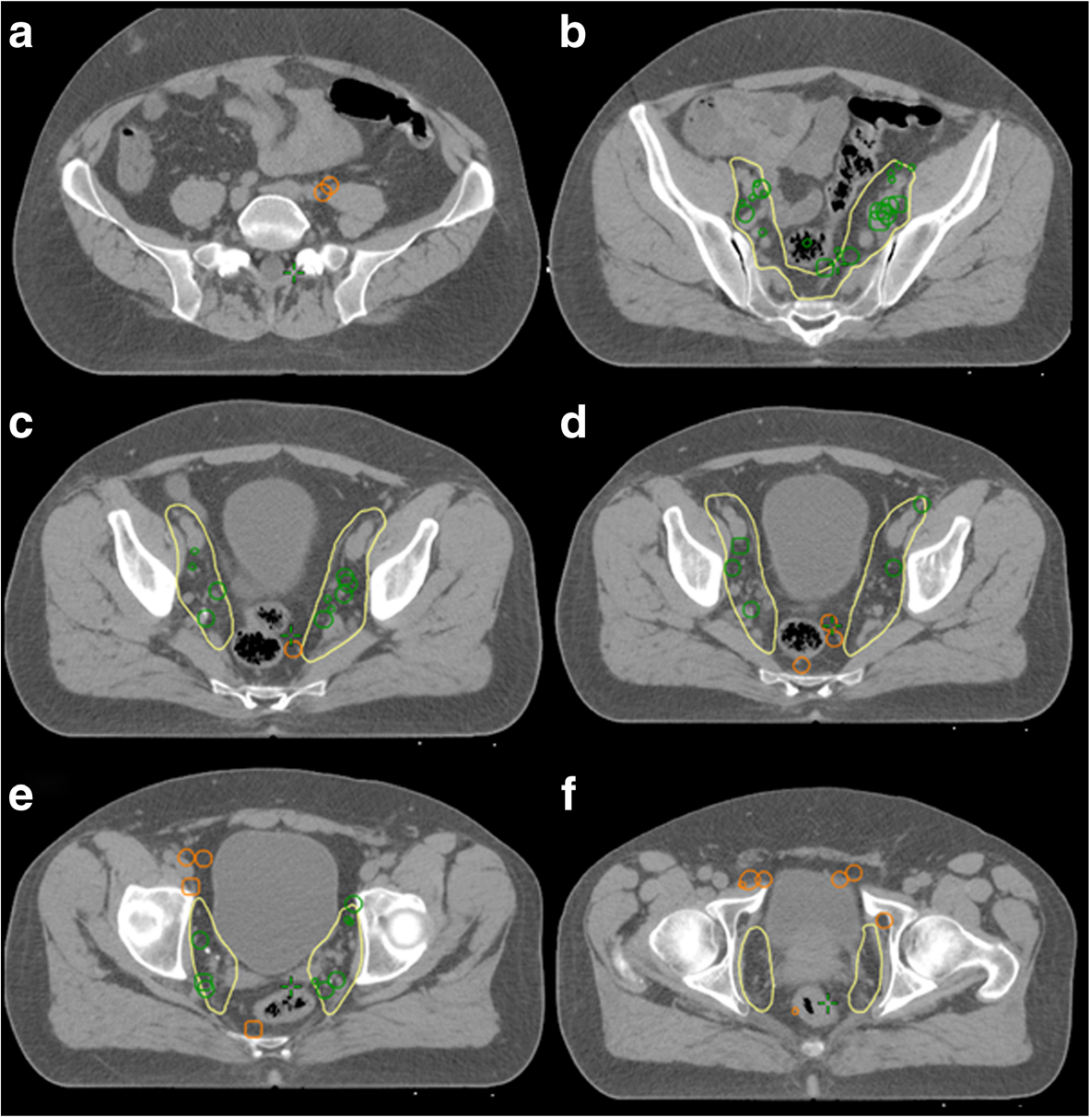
Images (a-f): Several slices of color-coded lymph nodes detected by PSMA-PET imaging that would have been inside (green) or outside (orange) of a standard radiation clinical target volume by RTOG consensus (yellow line). (a) lymph nodes between common iliac vessel and M. psoas, (b) multiple lymph nodes in the external and internal iliacal lymph drainage, (c + d) several lymph nodes para-rectal and one pre-sacral, (e) lymph-nodes pre-sacral, pre-acetabular and para-vesicular (f) more lymph nodes pararectal, pre-acetabular and para-vesicular, as well as one uncommen lymph node site lateral of the M. obturatorius internus
